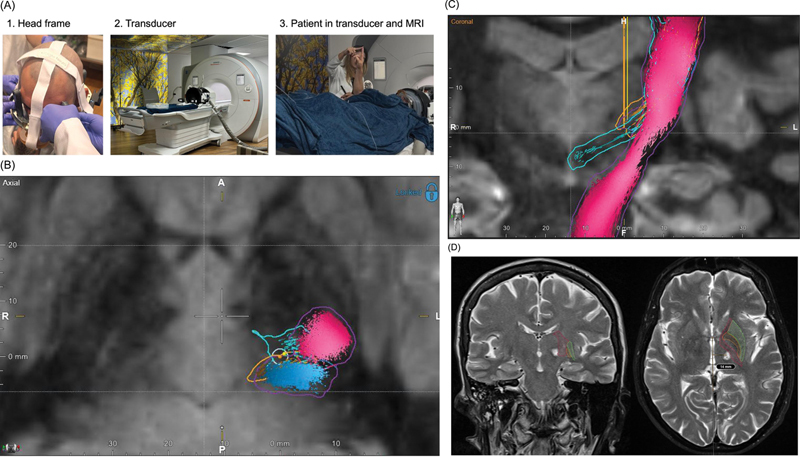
Abbreviations: DRTT, dentato-rubro-thalamic tracts; ET, essential tremor; FGATIR, fast gray matter acquisition t1 inversion recovery; HIFU, high-intensity focused ultrasound; MRI, magnetic resonance imaging; VIM, ventral intermediate nucleus of the thalamus.
